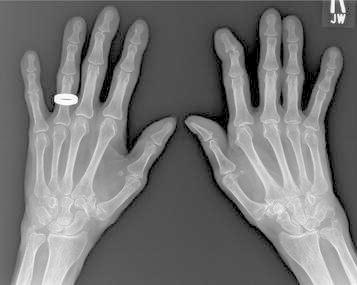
Radiographs of the hands showed erosions in the left third DIP and right second DIP, mild joint space narrowing with mild diffuse osteopenia, and erosions of the scaphoid bone with surrounding soft tissue swelling
